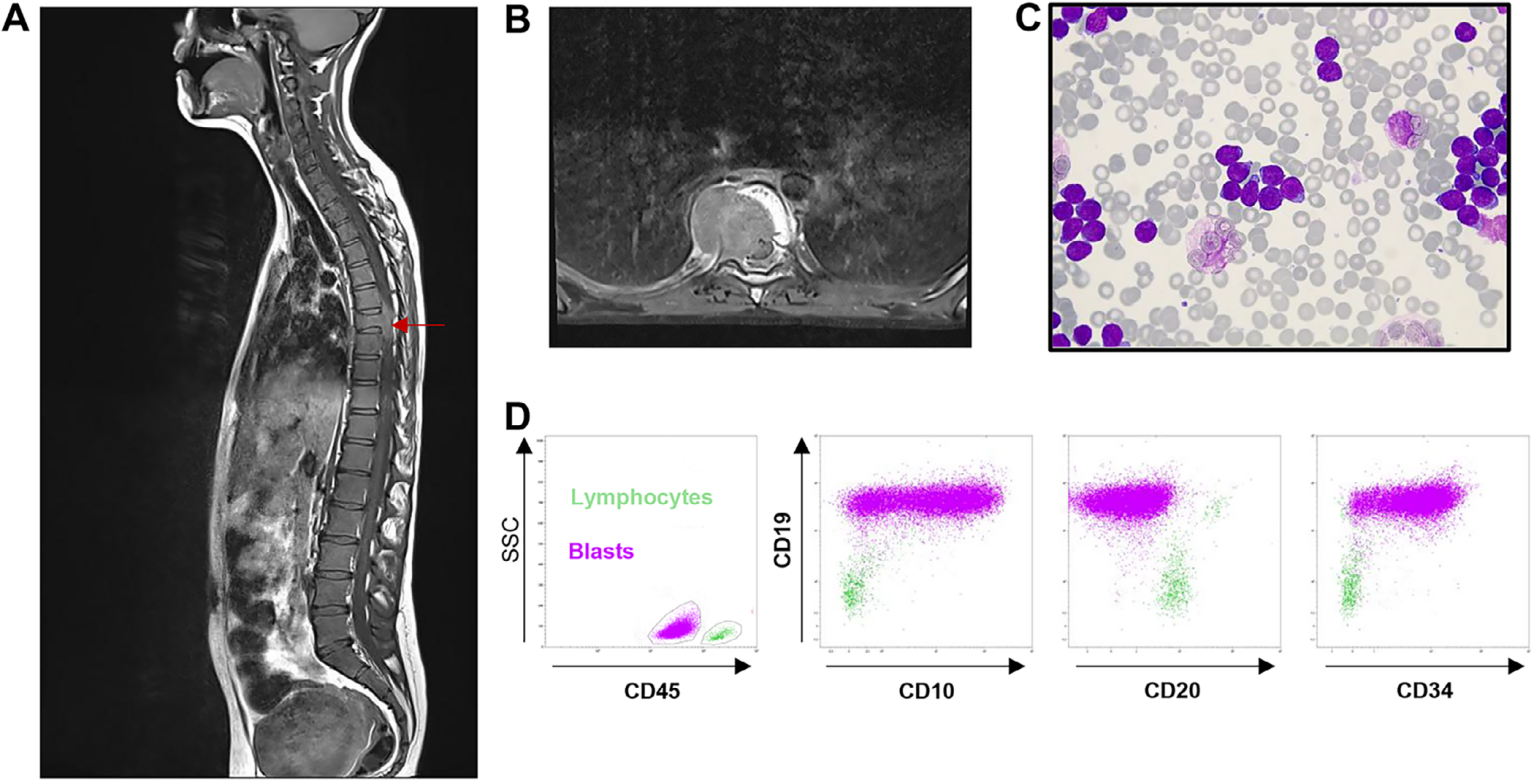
B‐ALL diagnosis with extramedullary lesions. (A, B) Sagittal T1 and axial T1 FS gadolinium MRI sequences showing right paravertebral mass of T7 (34 × 29 mm) with vertebral compression of T7, lytic lesion of the upper plateau of L1, diffuse medullary infiltration in hyposignal T1 of the various vertebral bodies. (C) (MGG, ×63) Lymphoblastic cells with high nuclear/cytoplasmic ratio, basophilic cytoplasm and immature chromatin. (D) Flow cytometry analysis of leukaemic cells and lymphocytes showing expression of CD10, CD19, CD20 and CD34 markers on the cell surface.
